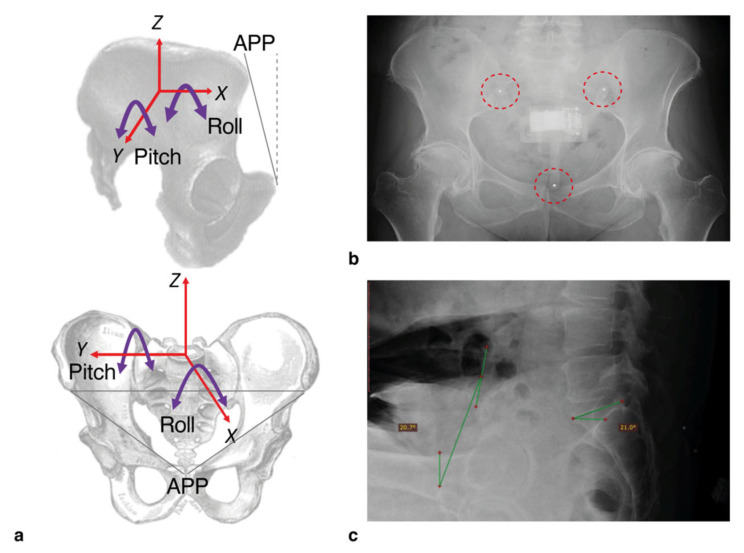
(a) The axes shown the image above demonstrate the measurements that can be taken from the IMU device. For this study, the only IMU measurement taken was pitch (i.e., tilt in the anterior-posterior direction, around the *Y*-axis). (b) The positioning of the device was checked by using 3 mm steel bearings inserted into the legs. The radiographic assessments were taken by measuring the angle of the anterior pelvic plane (defined as the plane between the anterior superior iliac spine and the pubic tubercle, relative to the vertical dashed line). (c) An example of this is shown.
